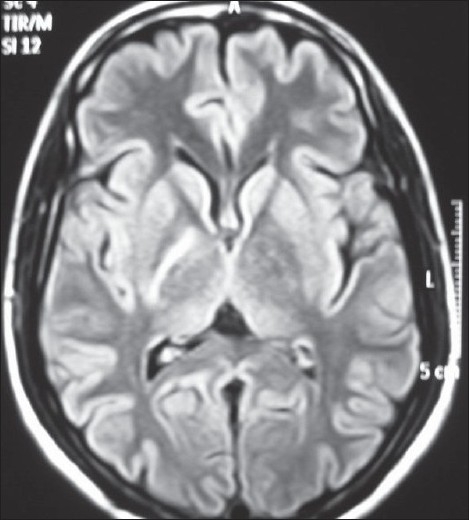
Axial FLAIR image showing hyperintensity of the posterior limb of the right internal capsule
